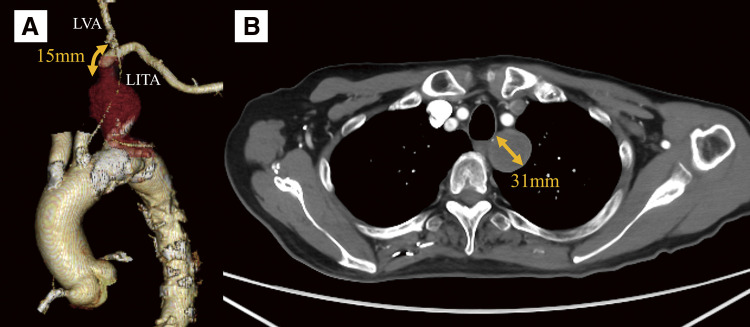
Fig. 1 Preoperative contrast-enhanced computed tomography images. (A) Three-dimensional reconstruction image showing the positional relationship between the left subclavian artery aneurysm, the left vertebral artery, and the left internal thoracic artery. To enhance the visualization of the left subclavian artery, the brachiocephalic artery and the left common carotid artery are rendered transparent. (B) Axial image. The left subclavian artery aneurysm is occluded by thrombus. LVA: left vertebral artery; LITA: left internal thoracic artery
